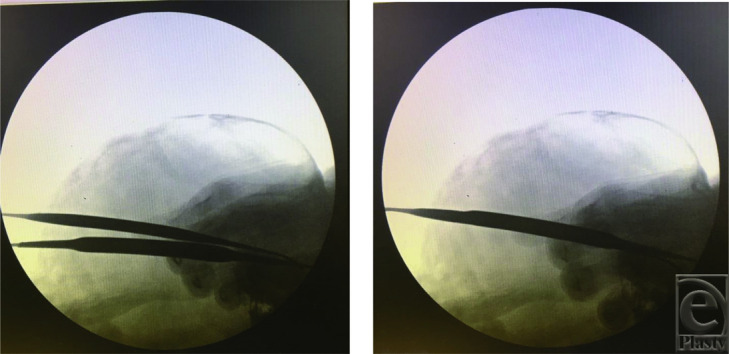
The superior and inferior osteotomy lines are marked using straight elevators under x-ray guidance. Elevators are adjusted (left) until they are parallel (right) before the osteotomy is done.
